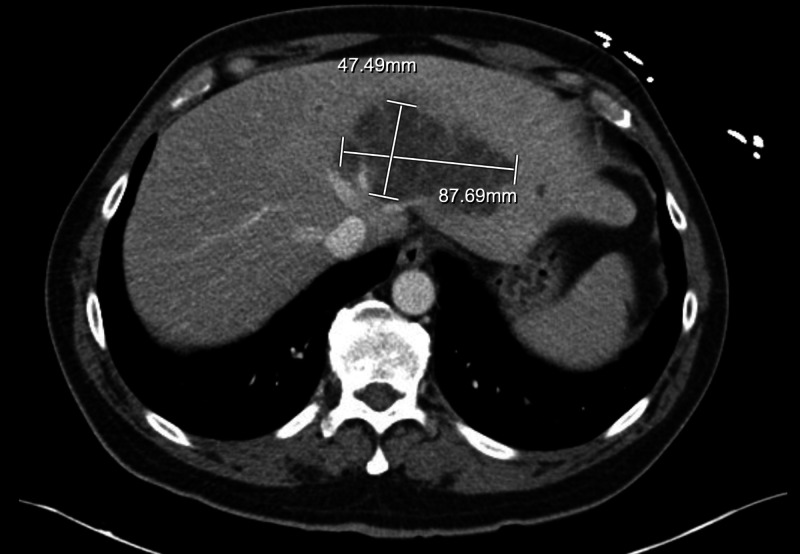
CT abdomen showing the largest multiloculated abscess (8 x 4 cm) in the left lobe of the liver.
